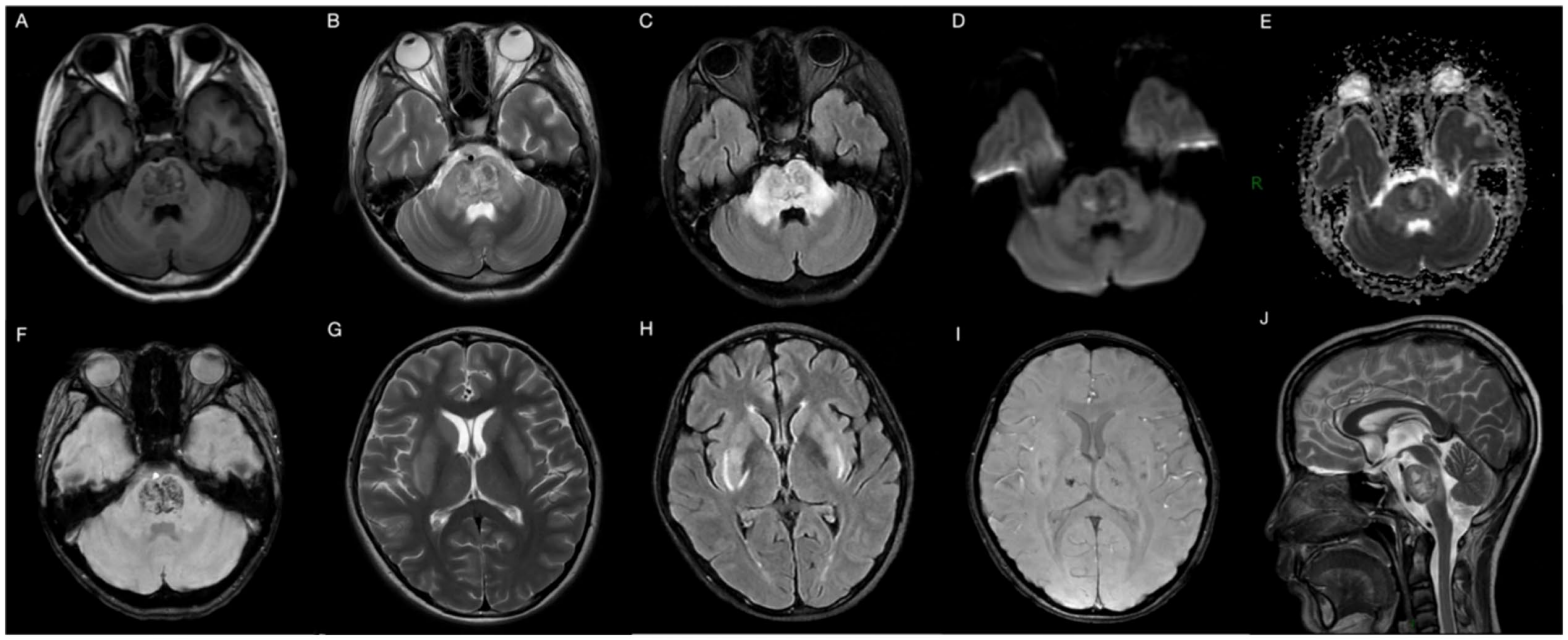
Brain MRI 29 days after ingestion showing abnormal heterogeneous intensities in the pons, bilateral brachium pontis, thalamus, caudate nucleus, putamen, posterior part of the external capsule, and posterior limb of the right internal capsule on T_1_WI (A), T_2_WI (B,G,J), T_2_-FLAIR (C,H), DWI (D), ADC-map (E), and SWI (F,I), in accordance with ODS. T_1_WI, T_1_-weighted imaging; T_2_WI, T_2_-weighted imaging; T_2_-FLAIR, T_2_-fluid-attenuated inversion recovery; DWI, diffusion-weighted imaging; ADC, apparent diffusion coefficient; SWI, susceptibility weighted imaging.
